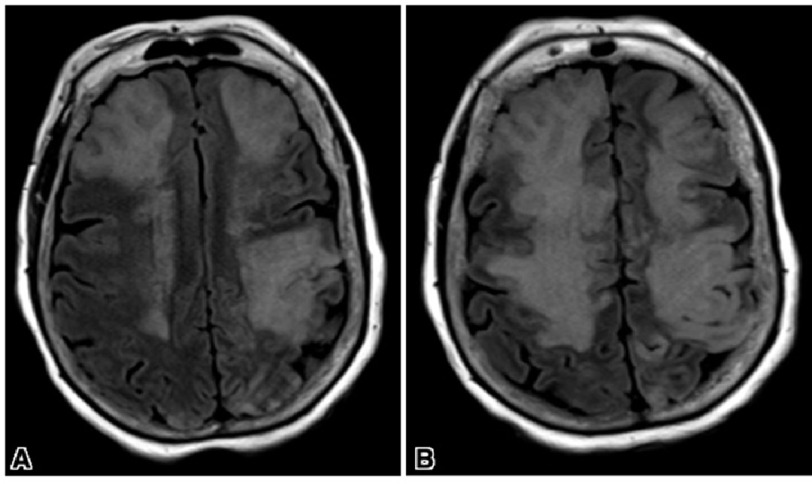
Follow-up brain MRI on day 3 showing the extensive progression of multiple areas of acute ischemic changes with the possibility of superimposed septic emboli with hemorrhage (A) with leptomeningeal involvement (B).
